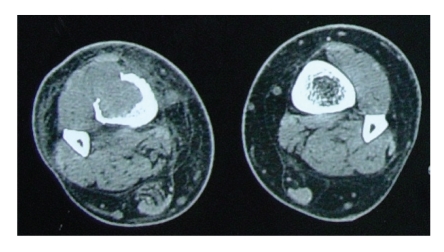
CT-scan of the tibia showing an intramedullar mass which destructs the cortical bone and spreads into the adjacent soft tissues.
